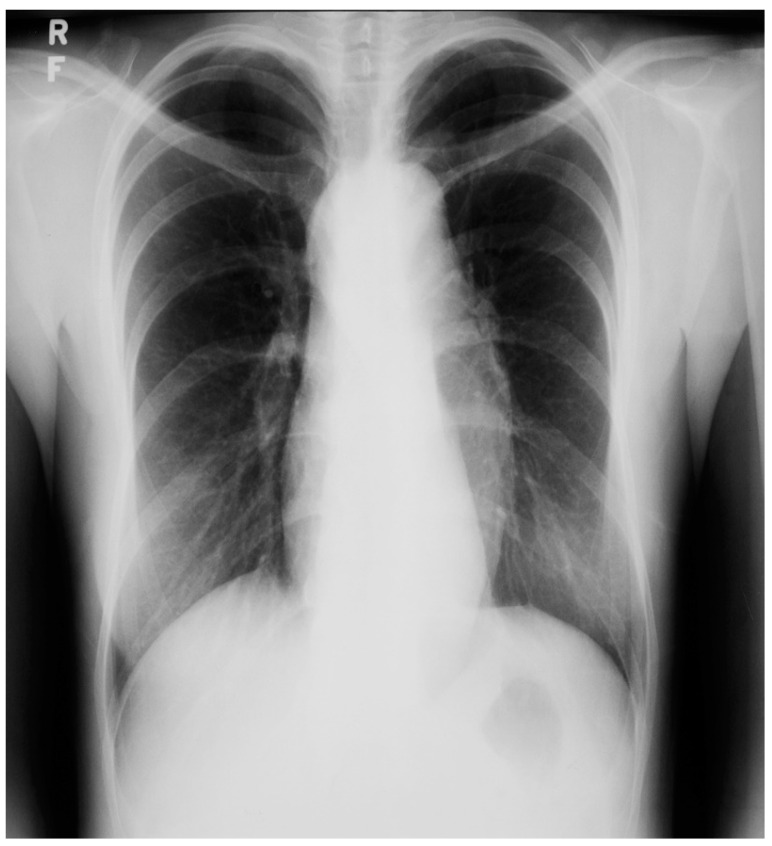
Posteroanterior chest X-ray of Marfan syndrome: This 24-year-old female with Marfan syndrome had a chest X-ray because of her tall, thin stature and hyperextensible joints. She was asymptomatic. The image is well centered and the inspiration is good. Notice the widened upper posterior dorsal intercostal spaces. This indicates an increased dorsal kyphosis. The C-T ratio is normal. The ascending aorta, aortic arch and descending aorta are dilated. There was a faint decrescendo diastolic murmur of aortic regurgitation at the right upper sternal border. The normal heart size suggests that, in this asymptomatic woman, the aortic regurgitation was mild.
